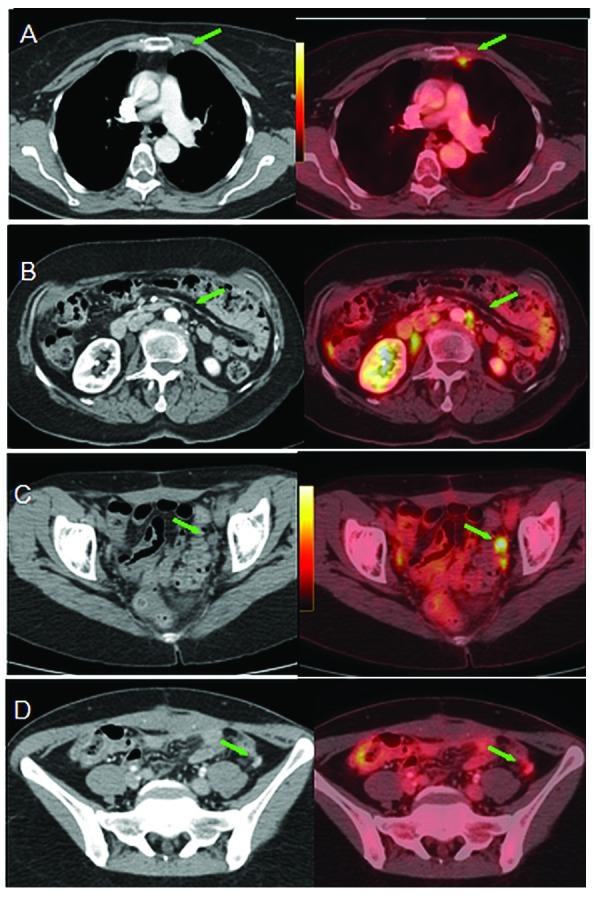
Unsuspected lesions detected with ^18^FDG PET/CT. The left column shows CT and the right column shows ^18^FDG PET/CT images. (A) Small hypermetabolic left internal mammary lymph node measuring 0.9 cm, with SUV=3.6 (green arrows). (B) Normal sized hypermetabolic abdominal paraaortic lymph node, <1.0 cm (green arrows). (C) Left iliac hypermetabolic lymph nodes (green arrows). (D) Small hypermetabolic implant in the left pelvic region, measuring 1.1 x 0.5 cm (SUV=1,5) (green arrows).
